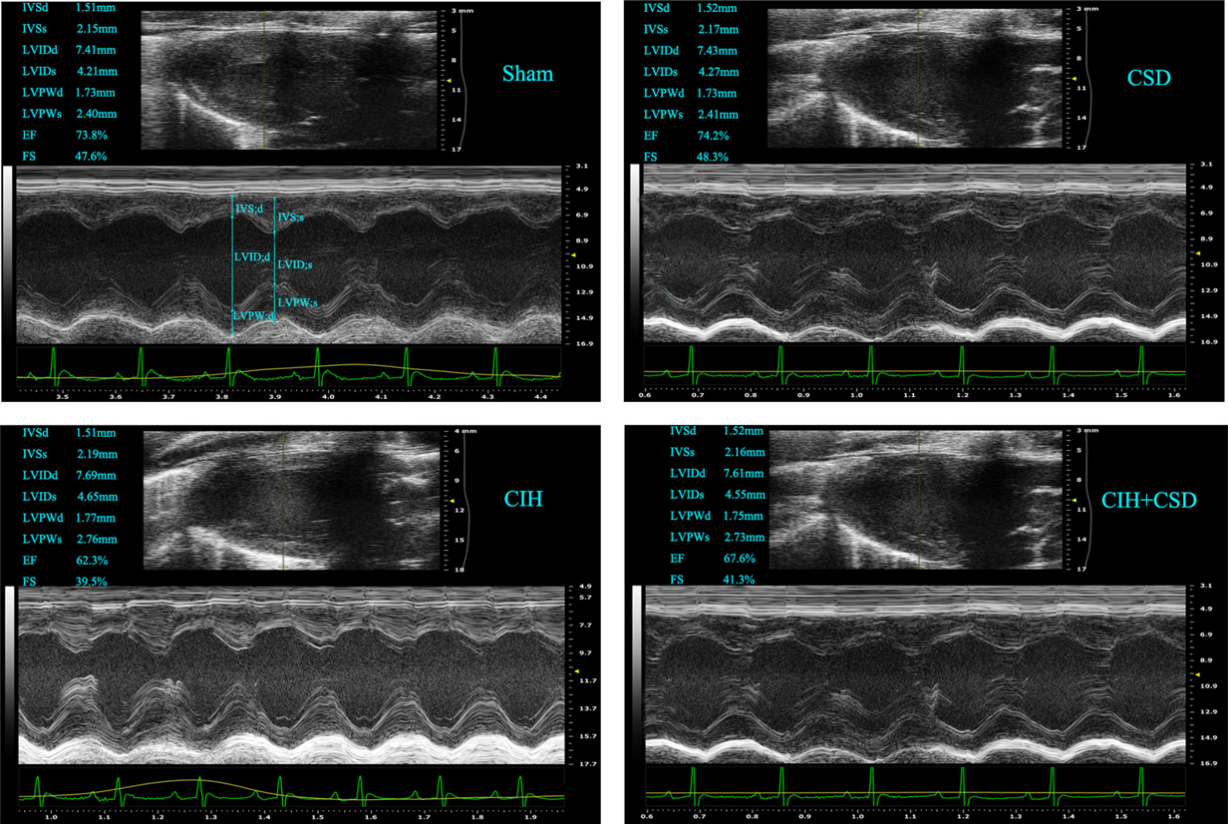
Ultrasonography shows the images of the 4 groups. CIH caused LV hypertrophy and remodeling. EF and FS were significantly decreased after CIH exposure, but CSD treatment increased the FS and EF. CIH indicates chronic intermittent hypoxia; CIH+CSD, chronic intermittent hypoxia with cardiac sympathetic denervation group; CSD, cardiac sympathetic denervation; EF, ejection fraction; FS, fractional shortening; IVSd, the intervernicular septum in diastole; IVSs, the intervernicular septum in systole; LV, left ventricle; LVIDd, left ventricular internal diameter in diastole; LVIDs, left ventricular internal diameter in systole; LVPWd, the left ventricle posterior wall in diastole; LVPWs, the left ventricle posterior wall in systole.
